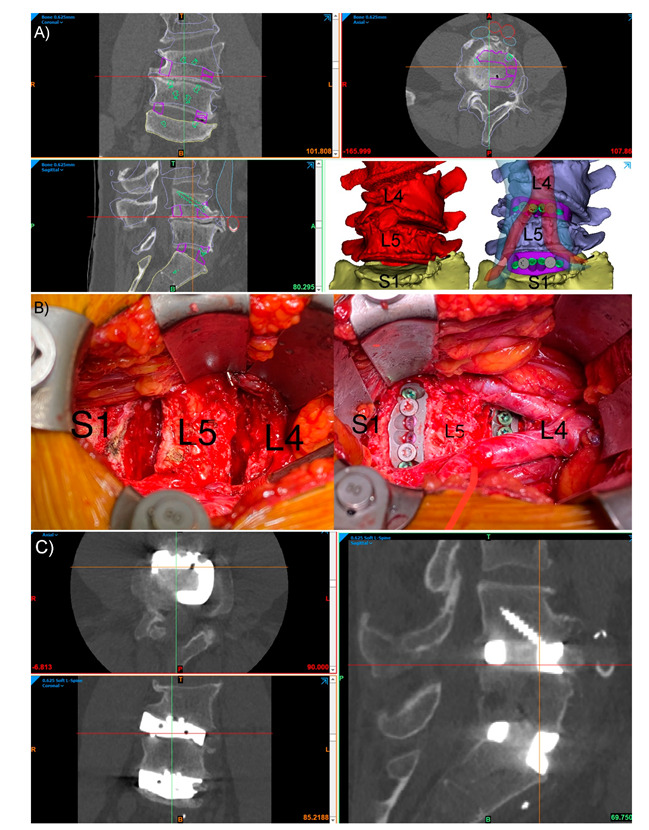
Integral screw fixation, stand-alone Anterior Lumbar Interbody Fusion (ALIF) patient-specific implants (PSIs) L4-5 and L5-S1, in an L4 congenital hemivertebra patient. (A) Preoperative CT with planned device (purple outlines), screws (green outlines), vertebral positions (blue outlines) and major vessels (inferior vena cava, blue, and aorta, red). The bottom right panel in (A) shows the preoperative pathological anatomy (red) and the planned postoperative state (blue) with translucent aorta (red) and inferior vena cava (blue) shown. (B) The intraoperative L4-L5 and L5-S1 discectomies (left) and final surgical reconstruction (right) with the aortic bifurcation at the L4 level shown. (C) Three-month postoperative CT of the construct showing good positioning of the devices, with no evidence of device migration or micromotion, and interbody fusion bone forming through the graft windows of both PSIs.
